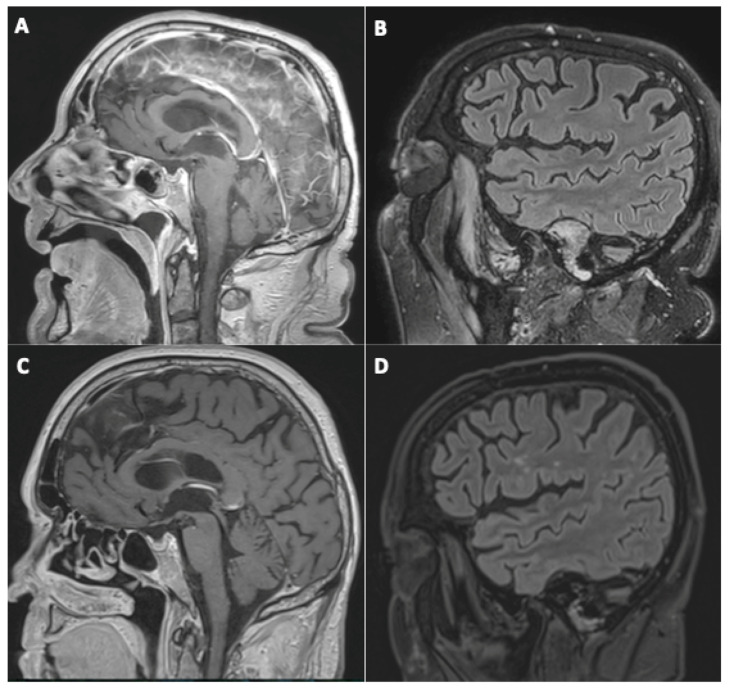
A 79-year-old man (#21) initially hospitalized (ICU) (A,B), who underwent a second MRI during follow-up 112 days later (C,D). Sagittal post-contrast three-dimensional T1-weighted spin-echo MR imaging (A,C) and sagittal FLAIR MR images (B,D). Basilar artery wall enhancement (A) with normalization of the vessel wall imaging during follow-up (C), and appearance of white matter FLAIR hyperintensities, presumed of a vascular origin (D).
